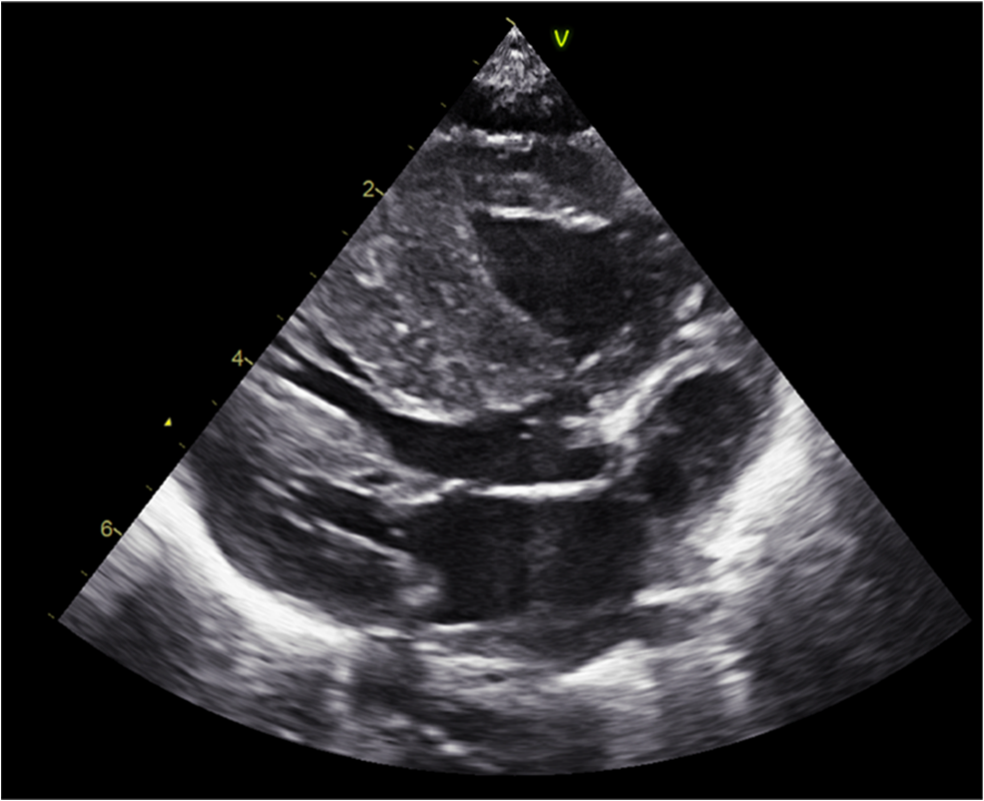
Echocardiographic images taken from a patient with congenital hyperinsulinism. The ultrasound features of the heart of patient with insulin-associated cardiac hypertrophy show specific thickening of the intraventricular septum
